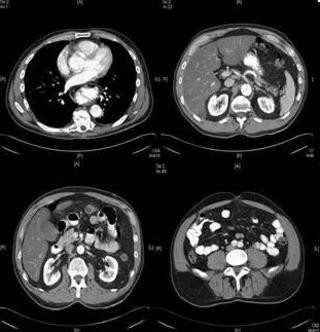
CT scans done on admission day; notice the aortic intimal flap in ascending aorta, descending thoracic aorta, abdominal aorta and common iliac arteries.
